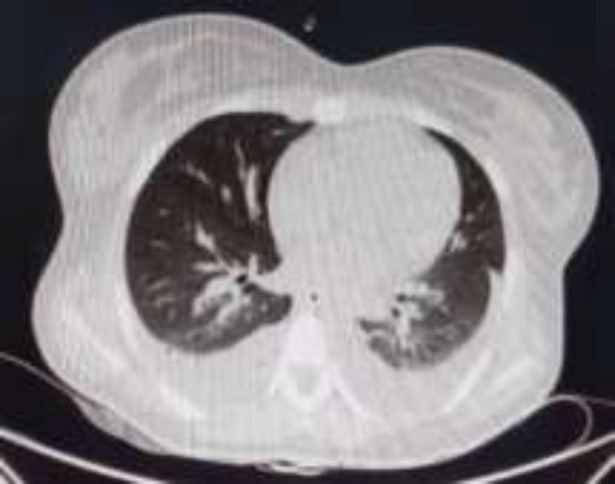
Chest cuts of abdominal CT scan revealed bilateral pleural effusion
